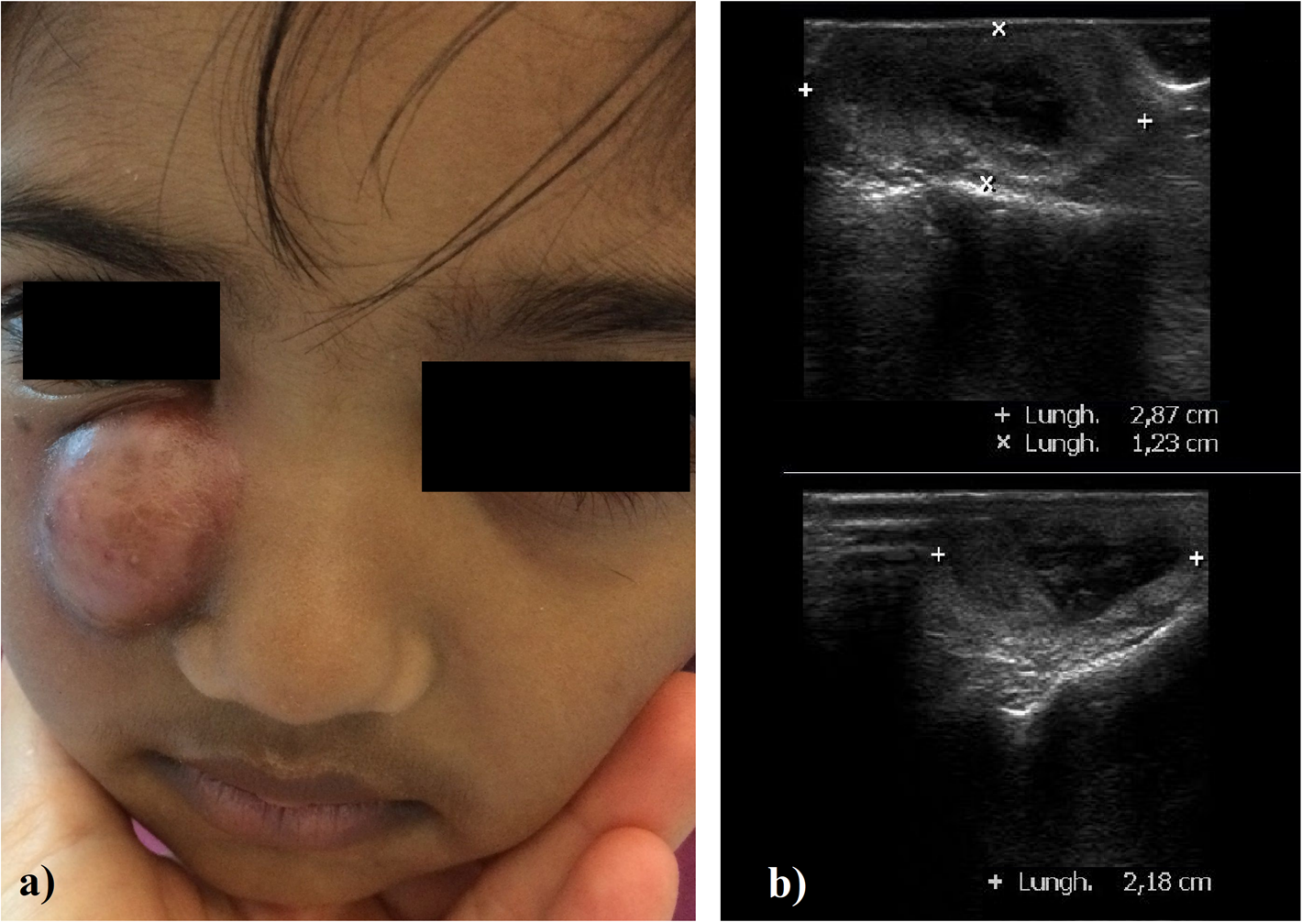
a Physical examaination at day 1: our patient had a voluminous and erythematous mass localized inferiorly to the medial canthus of the right eye, without purulent drainage or signs of conjunctivitis. b Ultrasound diagnostic aspects of the mass: a well-circumscribed round lesion filled by anechoic fluid is clearly detectable
